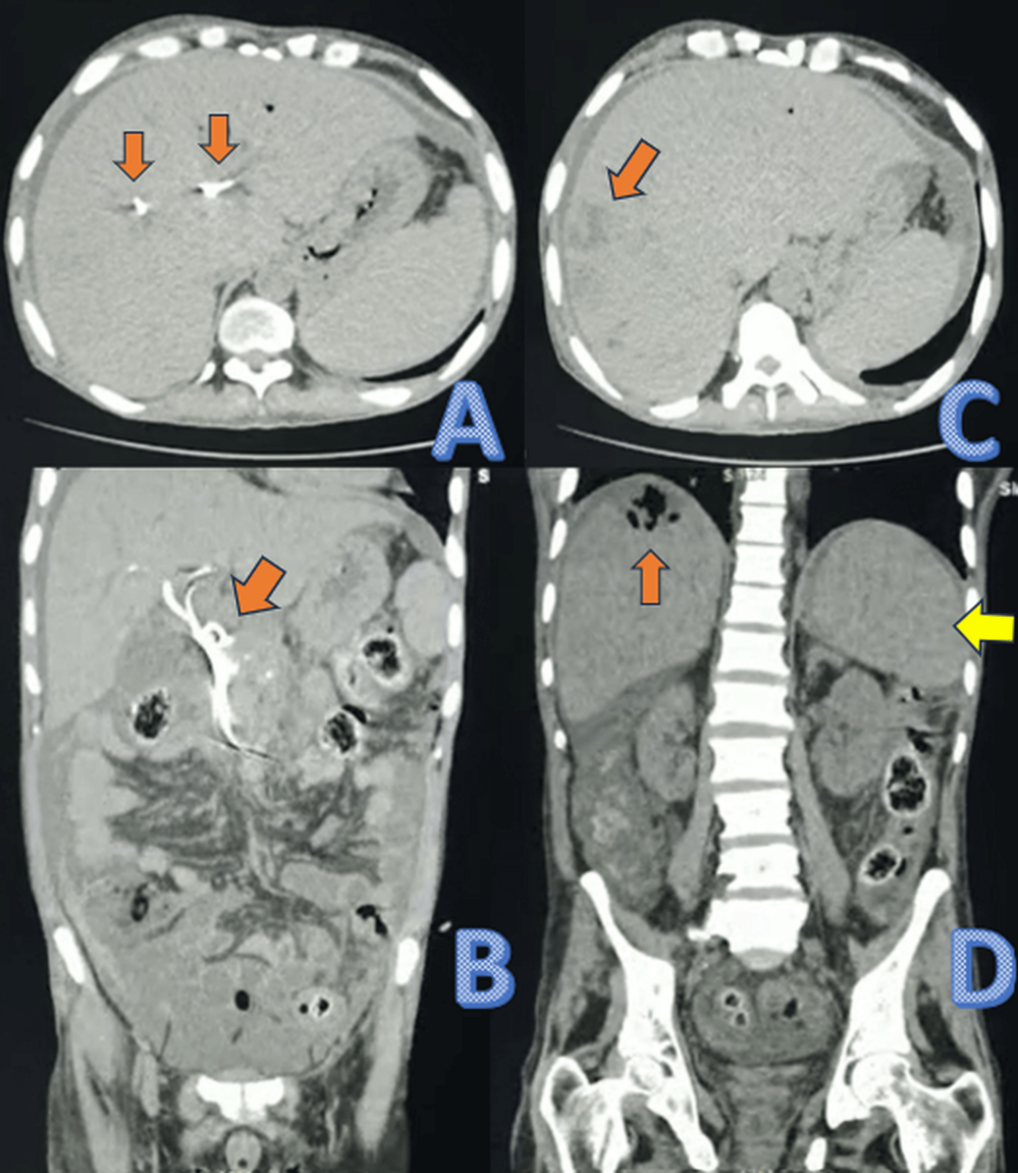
Non-contrast CT scan of the abdomen: (A) Axial CT scan showing biliary stents in situ. (B) Coronal reconstruction highlighting a biliary stent in situ. (C) Axial CT image revealing a hypodense lesion (orange arrow) suggestive of cholangiolar abscesses in the right lobe of the liver. (D) A coronal CT scan showing an orange arrow pointing to cholangiolar abscesses in the right lobe of the liver and a yellow arrow indicating an enlarged spleen CT: computed tomography
